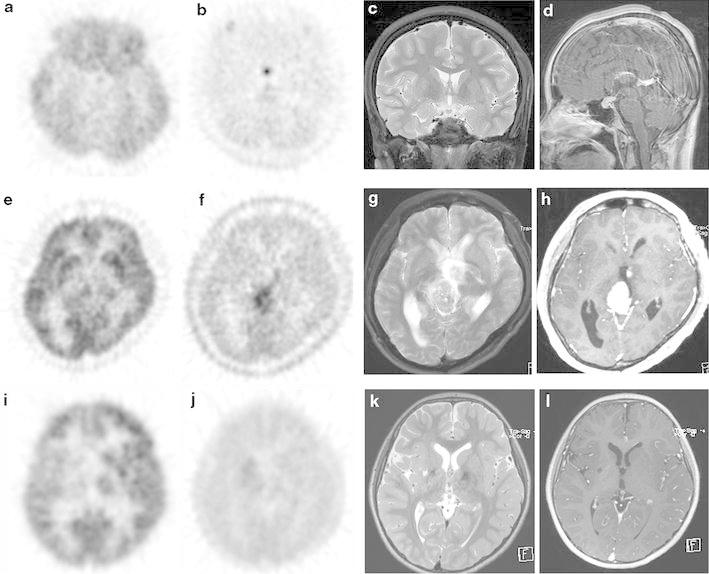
Representative case of CNS germinoma in the suprasellar region (No. 6). a FDG-PET detected slight FDG uptake. b MET-PET detected strong MET uptake. c, d T2-weighted images showing a small mass in the suprasellar region, and sagittal gadolinium-enhanced T1-weighted images showing subtle contrast enhancement. Representative case of a CNS germinoma in the pineal region (No. 9). e The tumor demonstrated an inhomogeneous uptake pattern, and the region displaying the strongest FDG-PET accumulation exhibited a similar uptake level to the gray matter. f MET-PET detected strong MET uptake. g, h T2-weighted images showing a mass in the pineal gland that exhibited inhomogeneous intensity on T2-weighted images, and gadolinium-enhanced T1-weighted images displaying strong enhancement. A suprasellar mass was simultaneously found in this case. Representative case of a CNS germinoma in the basal ganglia region (No. 4). i FDG-PET detected reduced FDG uptake in the right basal ganglia compared with the contralateral basal ganglia, and the FDG uptake of the tumor was intermediate between those of the normal white and gray matter. j The tumor demonstrated slight MET uptake on MET-PET. k T2-weighted images showing a region of increased signal intensity in the right basal ganglia, partial cystic changes, and right cerebral hemiatrophy. l On gadolinium-enhanced T1-weighted images, the tumor displayed very slight enhancement
